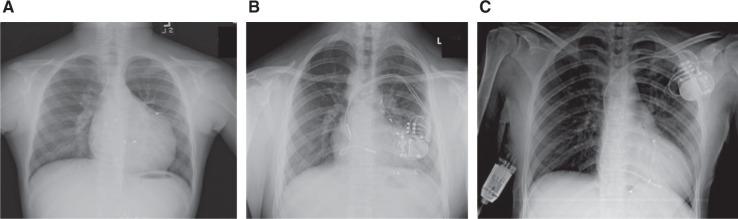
Chest X-rays showing variations of configurations for CRT in pediatric patients. A: Epicardial configuration. B: Transvenous configuration. C: Hybrid approach with LV lead tunneled from the epicardium.
